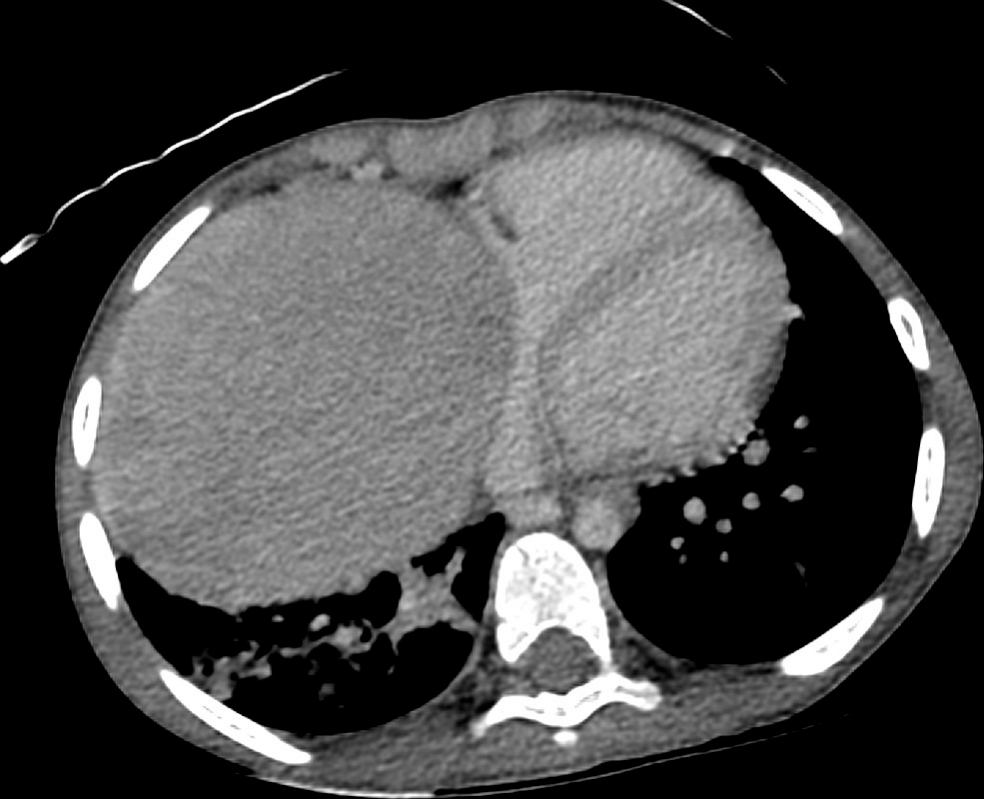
CECT of the chest showing the large anterior mediastinal mass, inseparable from the right-side cardiac border and atelectatic changes in the right lower lung lobe. CECT, contrast-enhanced CT.
